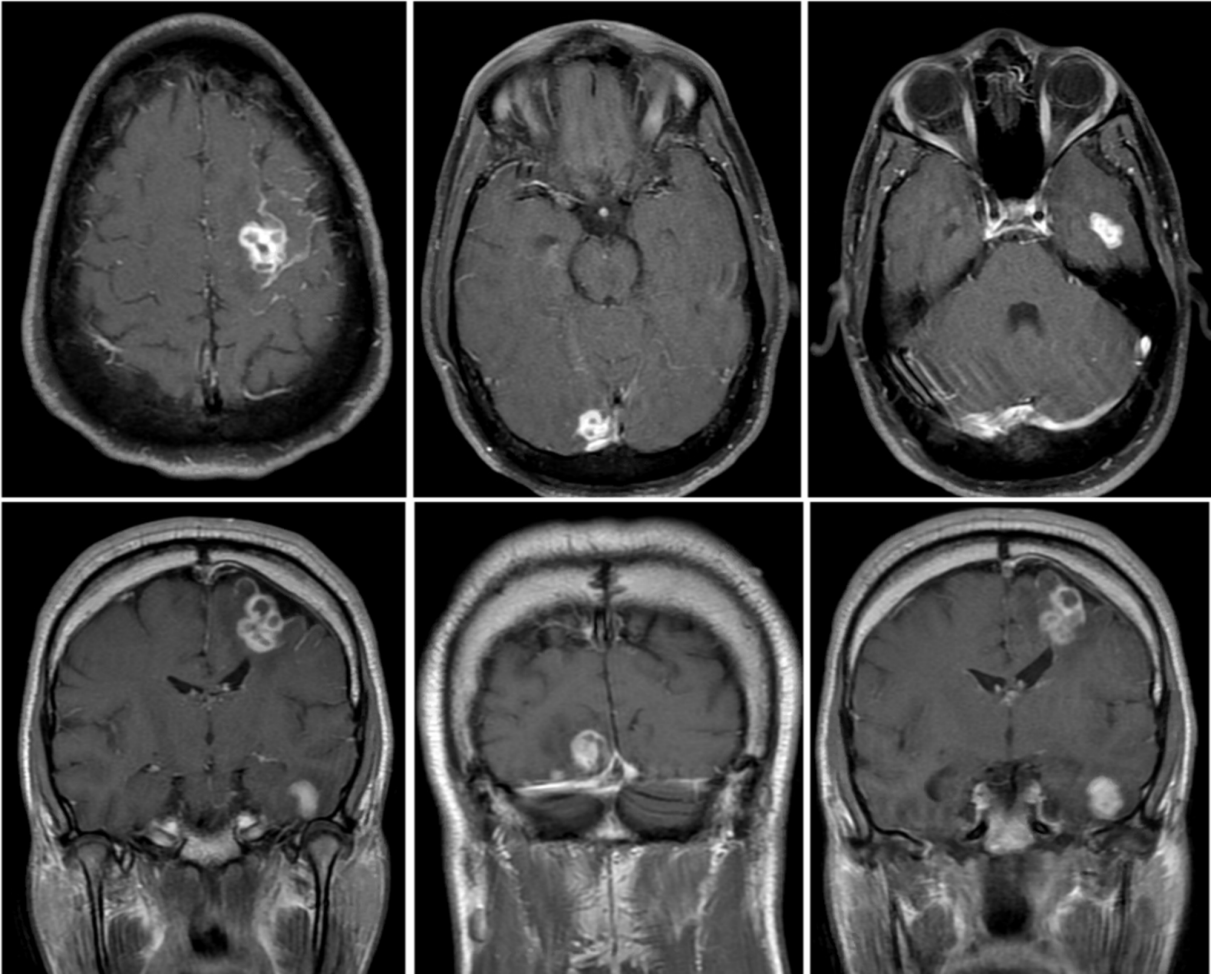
Brain MRI demonstrates multifocal enhancing lesions concerning for metastasis versus infection or abscesses. Enhancing lesions in the left superior frontal lobe (2.0 x 1.9 x 2.4 cm), left temporal lobe (1.7 x 1.3 x 1.7 cm), and right inferior occipital lobe (1.3 x 1.4 x 1.7 cm) with adjacent T2 hyperintense signal abnormality and mild perilesional mass effect. Lesions demonstrate faint peripheral diffusion restriction, without central diffusion restriction.
